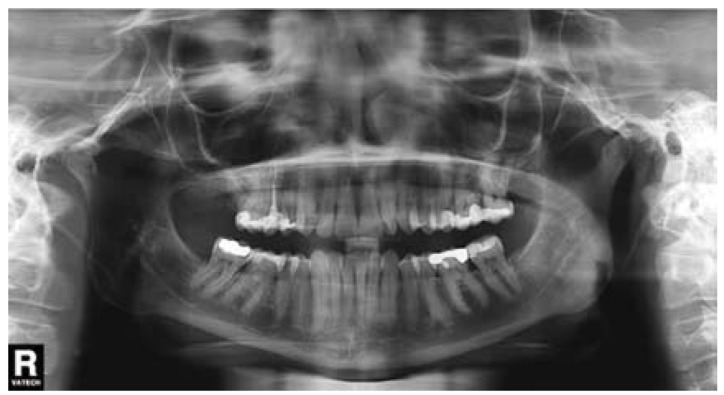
Panoramic radiograph revealed widening of periodontal ligament space in the root apex of the right mandibular second premolar.
